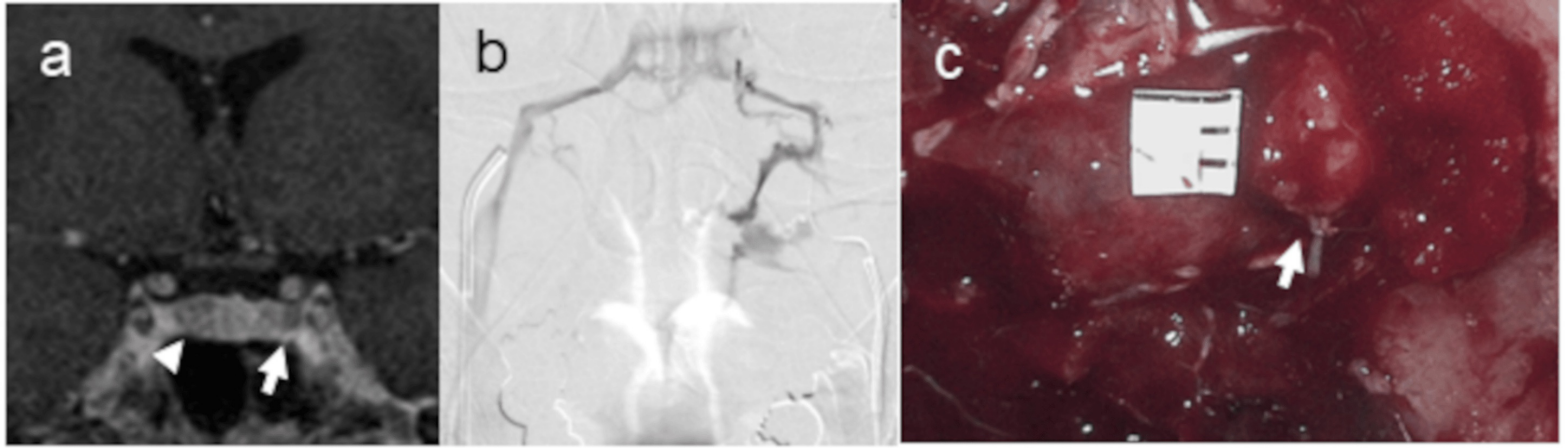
Case 2. A patient in whom venous drainage led to false-lateralization on IPSS, which was not corrected by prolactin adjustment (Table 2). A 40-year-old woman. An SPGR MR image shows equivocal lesions on the left side (arrow) as well as right side (arrowhead) (a). Venography from the left IPS demonstrated dominant venous drainage from the left to the right side (b). Intraoperative image demonstrates tumor located in the left lateral wing (arrow) (c). IPSS: inferior petrosal sinus sampling, SPGR: spoiled gradient echo sequence
